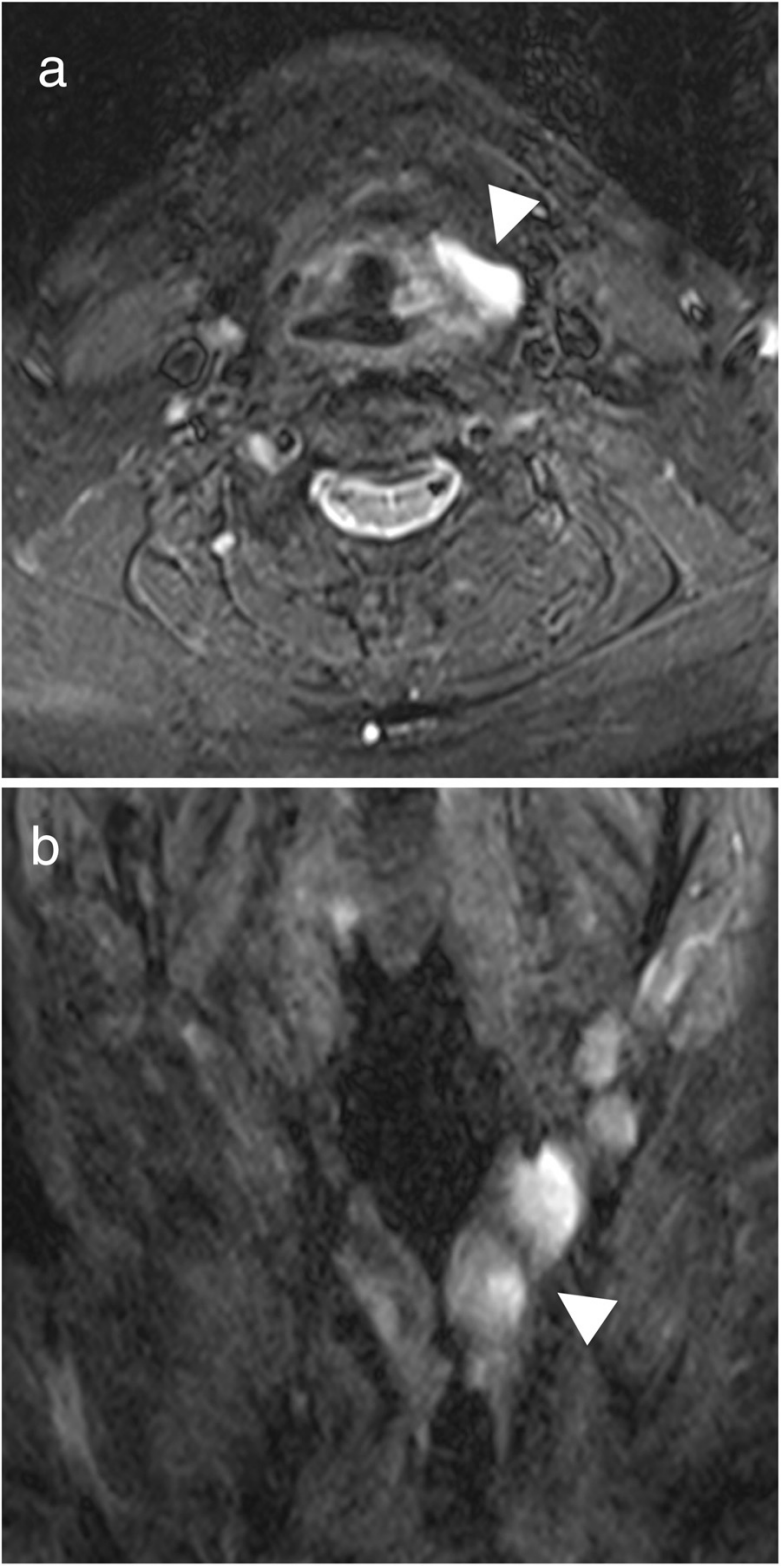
Fat-suppressed T2 weighted MRI of the larynx. Multi-cystic hyperintense lesion occupying the left supraglottic region (arrowhead). a) axial, b) coronal
